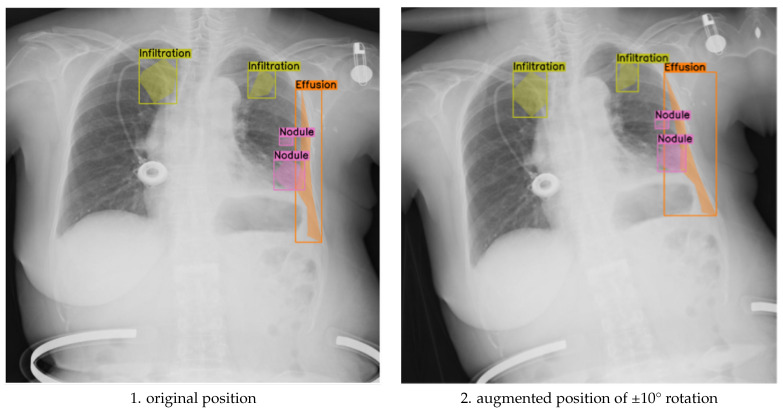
Comparison of original and discrete rotations at (−10°, +10°) augmented chest X-ray images.
